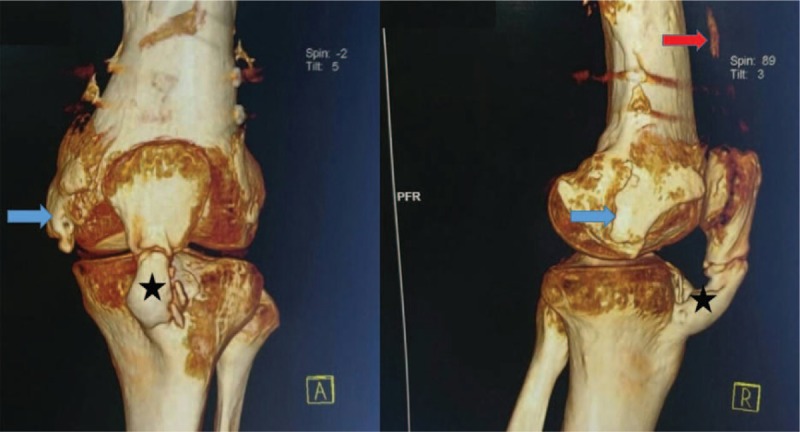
The computed tomography (CT) showed ossification of the patellar tendon (black star), quadriceps tendon ossification (red arrow), and medial collateral ligament (blue arrow).
